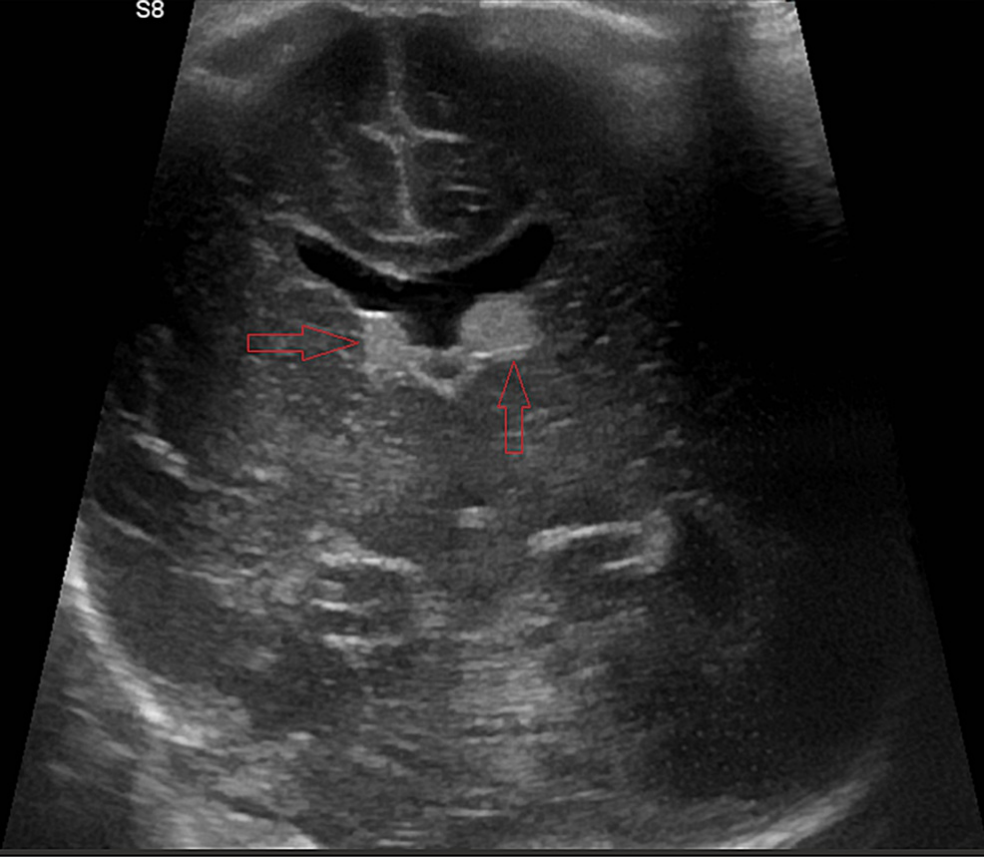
Neurosonography from the final day of life displays bilateral hematomas in the caudothalamic grooves (arrows)
